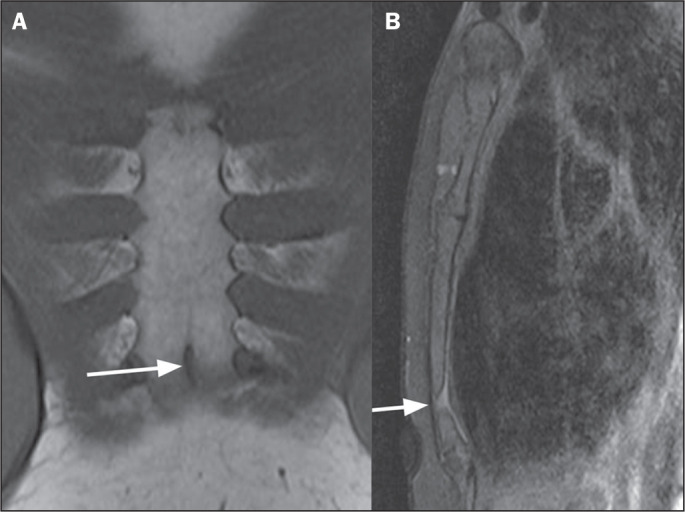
Sternal foramen. A: Coronal T1-weighted MRI of the sternum with fat
